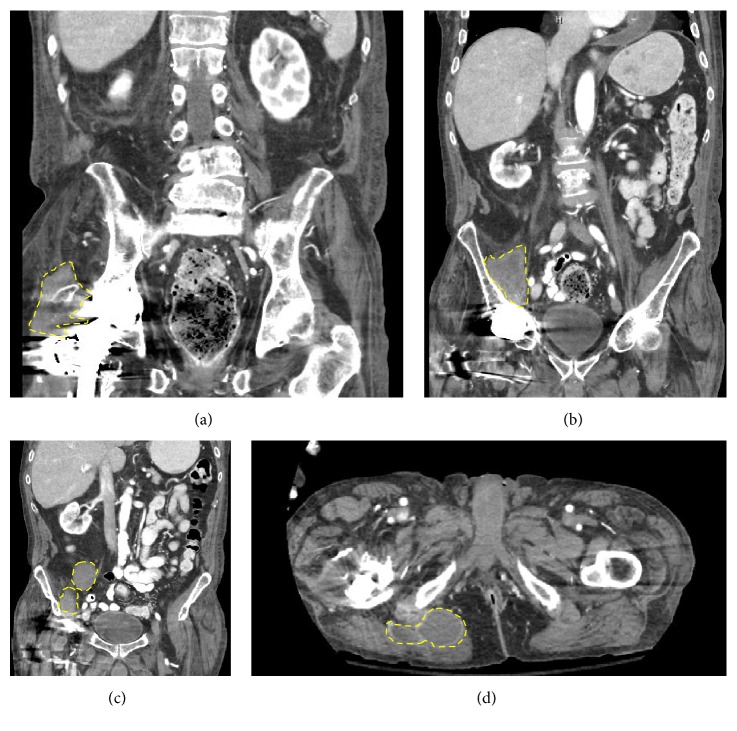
CT abdomen. (a) Coronal cross section demonstrating a right hip complex fluid collection. (b) Right posterior iliac fossa fluid collection. (c) Right anterior iliac fossa fluid collections. (d) Transverse section demonstrating right buttock fluid collection.
